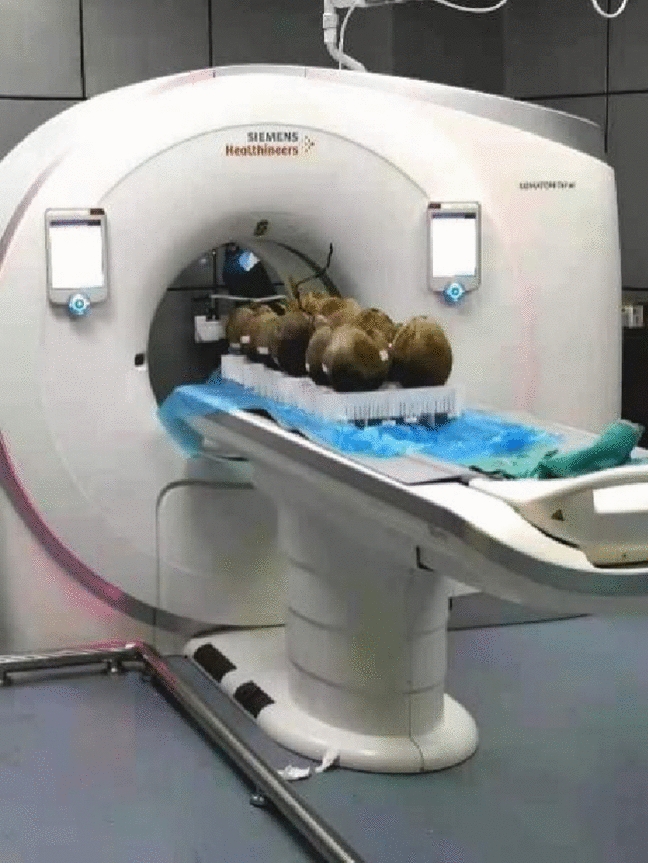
CT machine scans coconut samples
